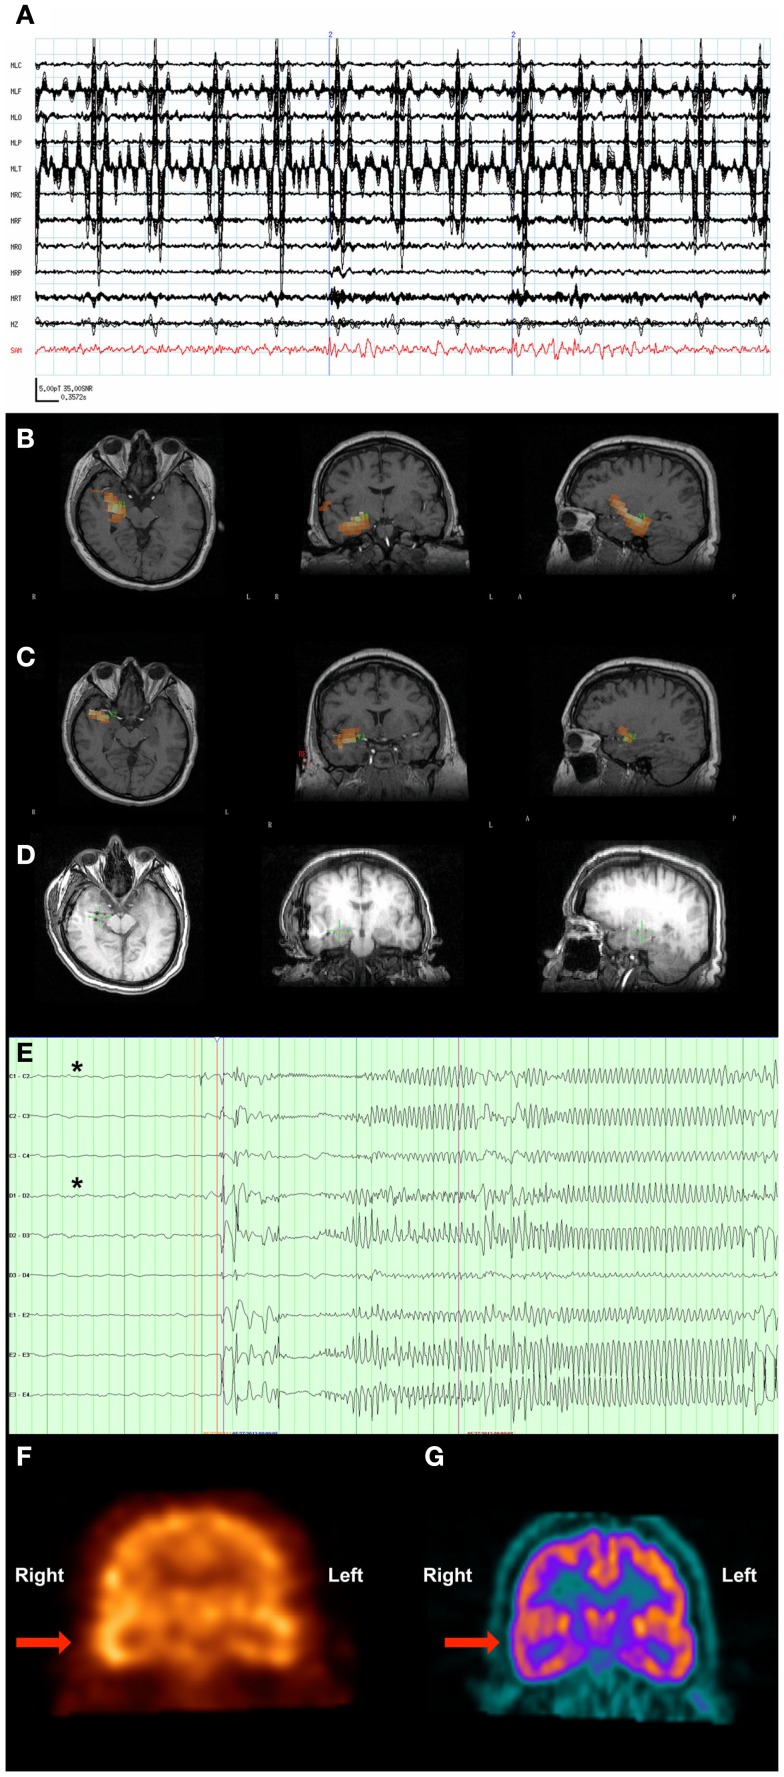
Patient 3. (A) The raw MEG sensor data (black traces) exhibited strong artifacts due the patient’s VNS, but the source signal reconstruction (red) from the amygdala displayed clear spikes. (B) The SAM(g2) statistical parametric maps indicated a right hippocampal focus as well as (C) another focus in the amygdala. (D) A CT scan reveals the placement of the hippocampal and amydalar depth electrodes, as well as the location of the hippocampal focus (green cross) as identified by SAM(g2). (E) An example of a seizure that arose from the anterior hippocampal and amygdalar electrodes, (black asterisks). (F) A coronal plane ictal SPECT image demonstrating hyperperfusion of the right temporal lobe (arrow). (G) A coronal plane ^18^F-FDG PET image demonstrating hypometabolism of the right temporal lobe (arrow).
